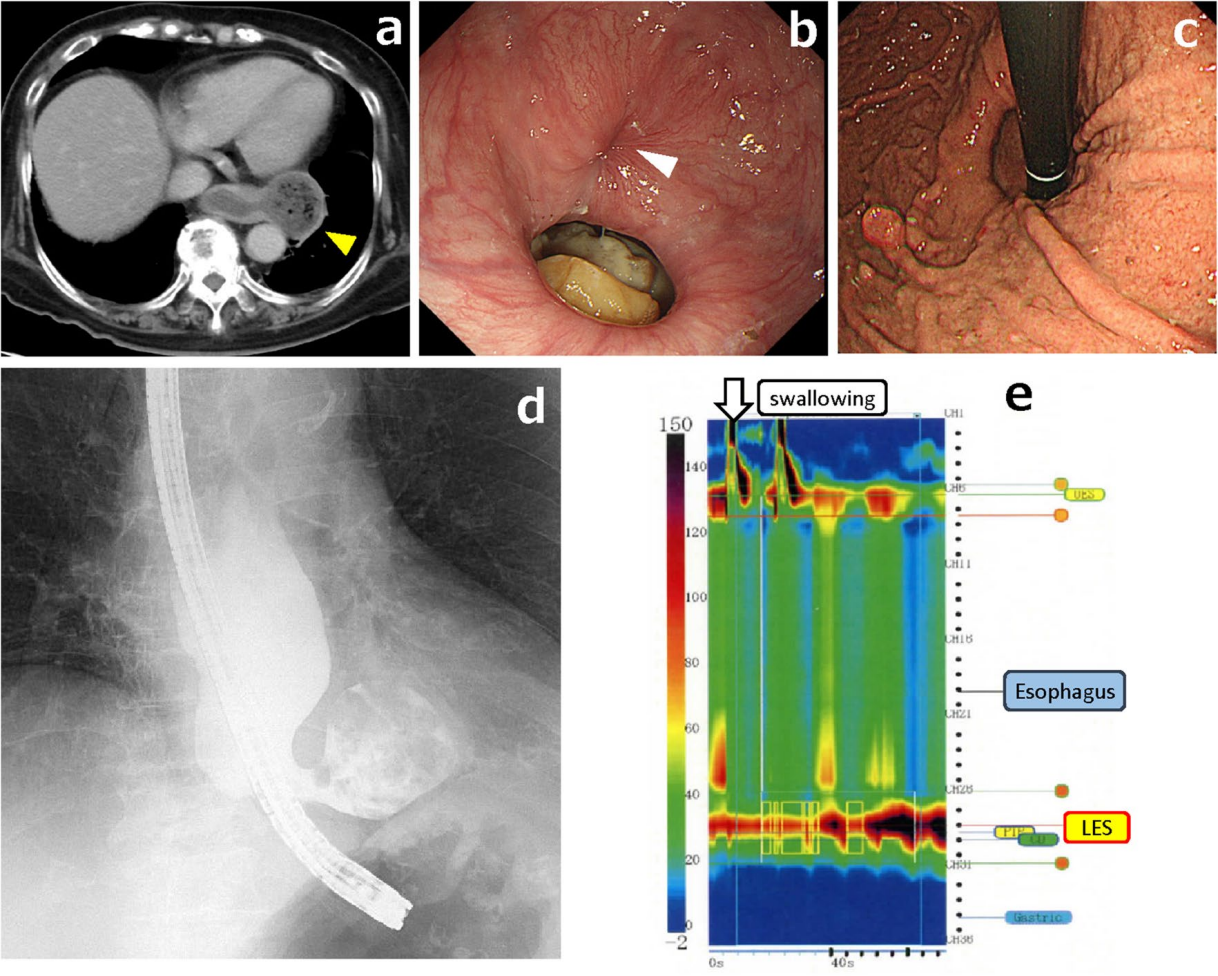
Results of preoperative examination. a Computed tomography revealed the epiphrenic diverticulum (ED) in the left thoracic cavity (*yellow arrowhead*). b Upper gastrointestinal endoscopy revealed the ED orifice near the esophagogastric junction (*white arrowhead*) and a food residue reservoir. c Paraesophageal hernia. d Barium esophagram revealed the ED of approximately 7.5 cm in size in the lower thoracic esophagus. e Esophageal manometry revealed a median integrated relaxation pressure of 54.6 mmHg, which was indicative of failure of lower esophageal sphincter (LES) relaxation, leading to a diagnosis of achalasia
